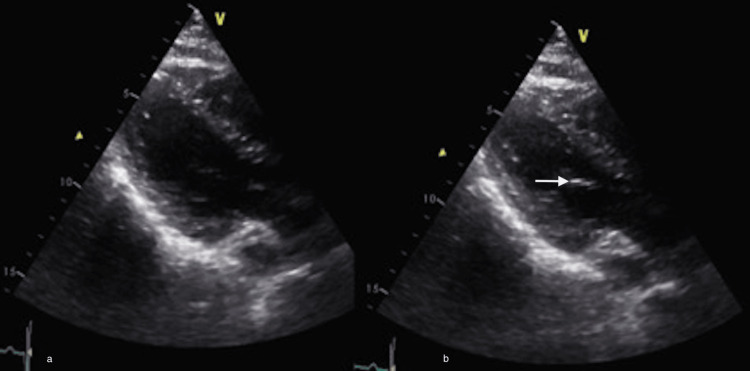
Transthoracic echocardiogram. (a) The image on the left shows the ventricle without hemodynamic alterations or changes in contractility. (b) On the right, in the subvalvular apparatus toward the interventricular septum, a hyperechoic image of 3 mm is observed (arrow).
